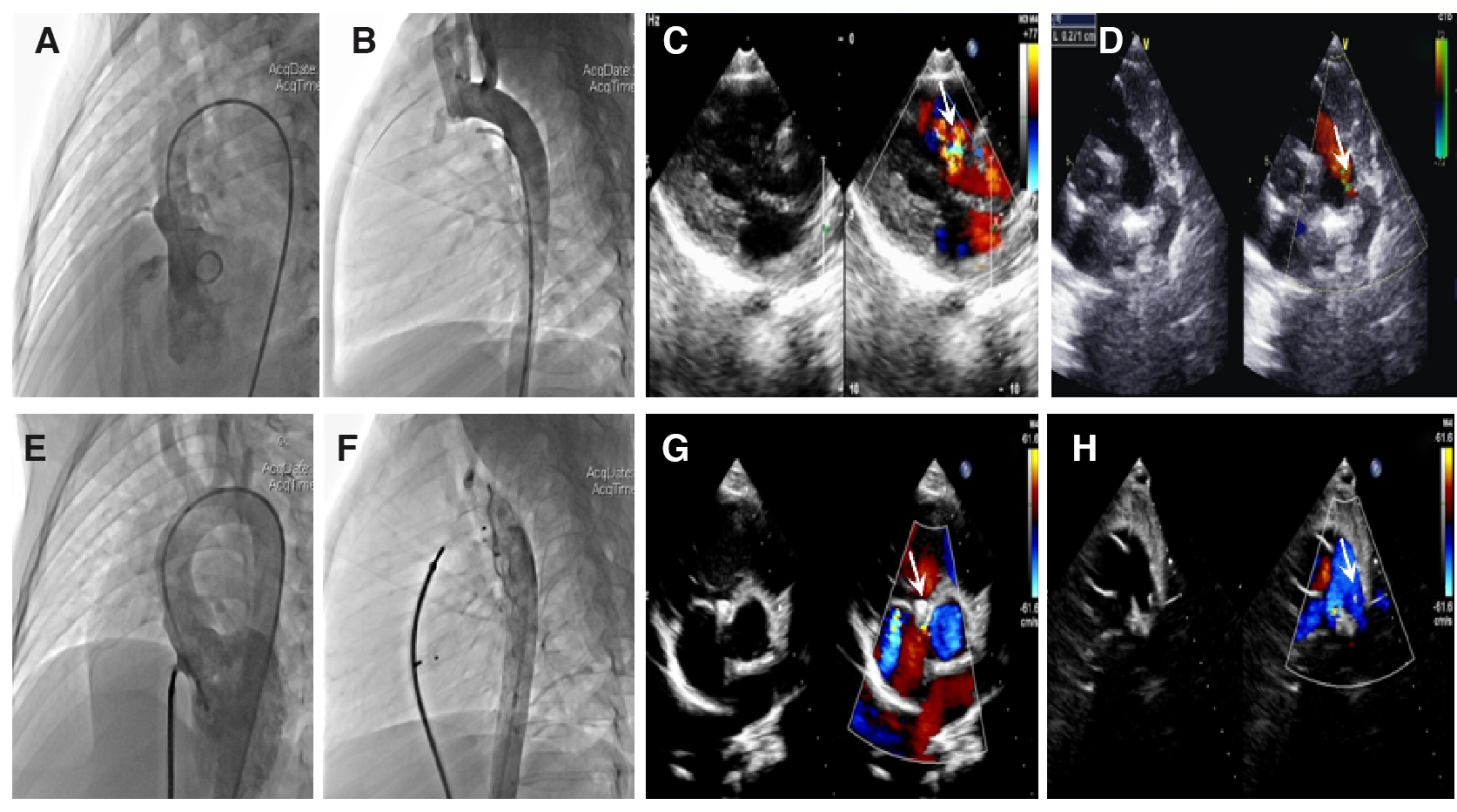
Angiocardiography and echocardiography images in a patient with ventricular septal defect (VSD) combined with patent ductus arteriosus (PDA). Preoperation: (A) Long-axis view of the left ventriculography showing a significant left-to-right shunt through the VSD. (B) Lateral view of the aortic angiography showing an evident shunt from the aorta to the pulmonary artery through the PDA. (C) Parasternal left ventricular long-axis view showing a left-to-right shunt passing through the VSD. (D) Parasternal short-axis view at the base of the main pulmonary artery (MPA) and its branches showing a shunt from the aorta to the pulmonary artery through the PDA. Postoperation: (E) No evident shunt is detected after VSD occlusion by left ventriculography. (F) No aortopulmonary shunt is detected. (G,H) Parasternal short-axis view showing that the VSD and PDA occluders are properly placed; no evident shunt is detected. The white arrow points to the defect and occluder.
